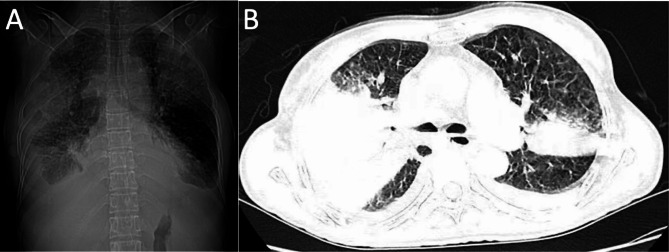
CT images prior to and after the treatment of the patient with the co-infection of H5N1 and COVID-19. The radiological examination of the patient after admission revealed an air bronchogram, lung consolidation, and bilateral pleural effusion (A and B)
